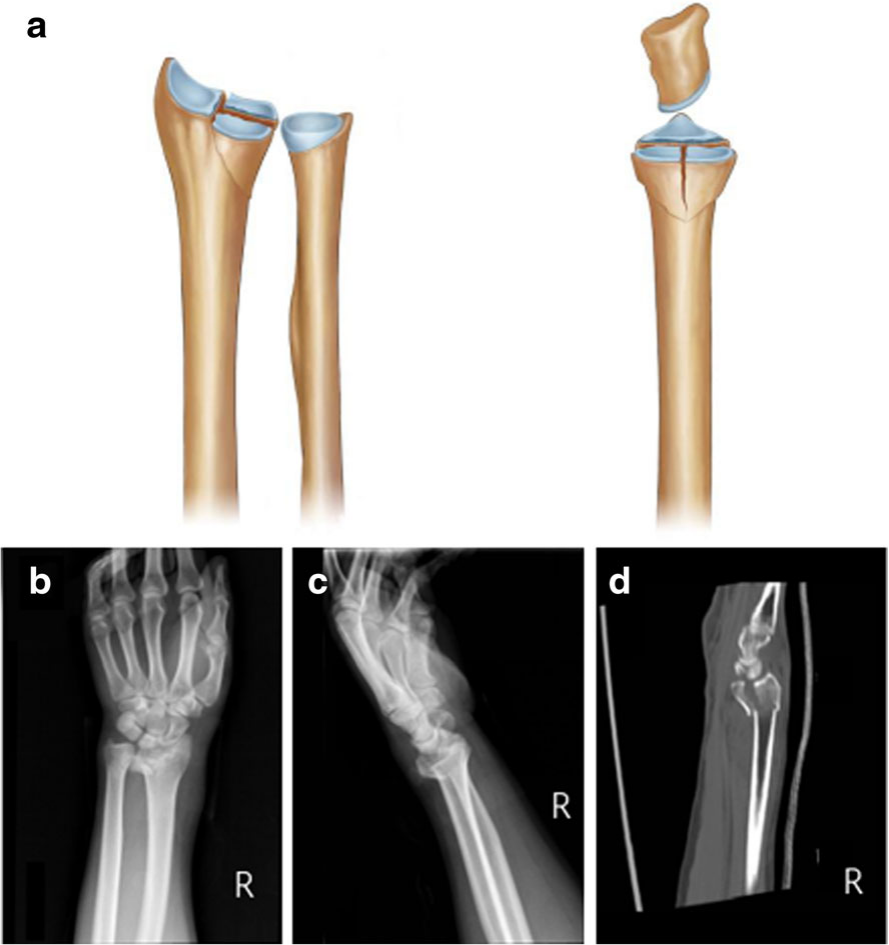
Double die-punch fracture. a The lunate fossa is split into two halves, each depressed toward the volar and dorsal directions, respectively (*left*, anterior view; *right*, lateral view). b–d Anteroposterior lateral radiograph and CT scans (right)
